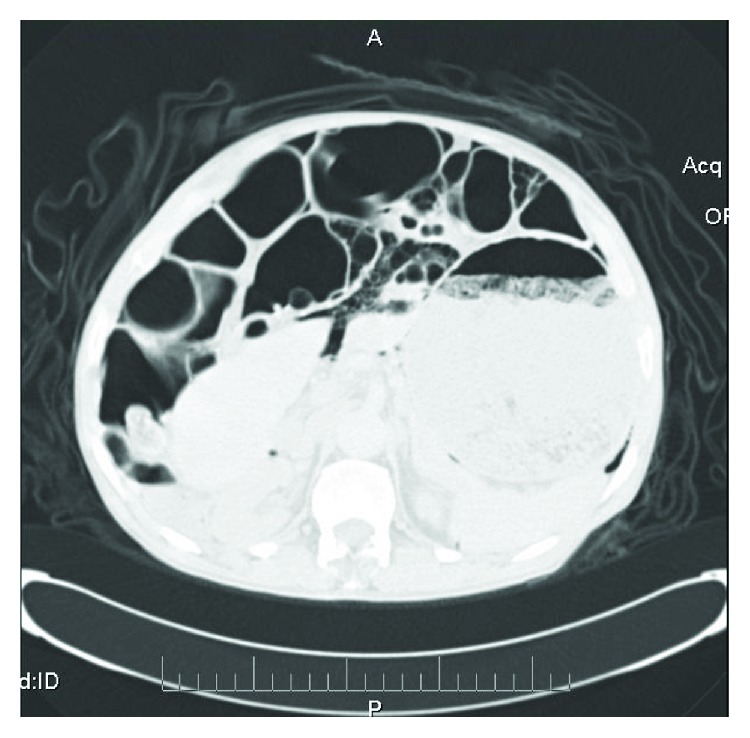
Abdominal CT scan (pulmonary window) reveals PCI.
